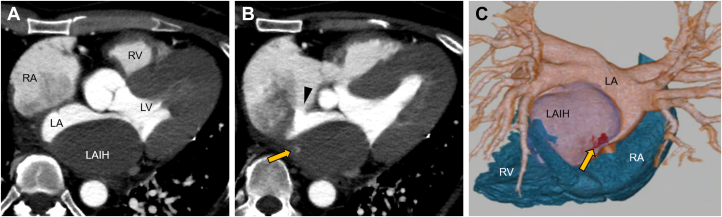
Cardiac CT of the Left Atrial Intramural Hematoma (A) Axis imaging of the LAIH. (B) Contrast extravasation into the LAIH (arrow). The black arrowhead indicates the iatrogenic atrial septal defect. (C) Three-dimensional reconstruction of cardiac CT posterior view showing the spatial relationship of the right heart, left atrium, pulmonary veins, and LAIH. The red portion (arrow) indicates contrast extravasation. CT = computed tomography; LA = left atrium; LAIH = left atrial intramural hematoma; LV = left ventricle; RA = right atrium; RV = right ventricle.
